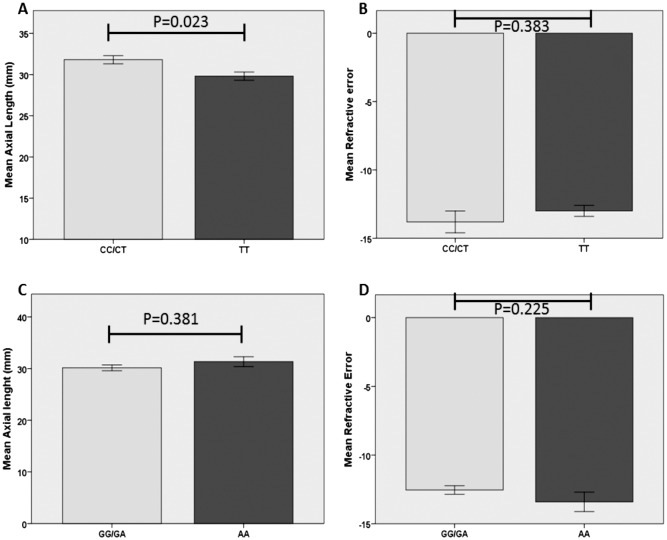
Axial length and refractive error of Col8A1 gene SNPs (rs13095226 and rs669676) genotypes. (A-B) Axial length showed statistically significant differences between CC/CT vs TT genotypes of rs13095226, whereas no differences were found for Refractive error. (C-D) Axial length and Refractive error (respectively) did not show differences between GG/GA vs AA genotypes of rs669676.
